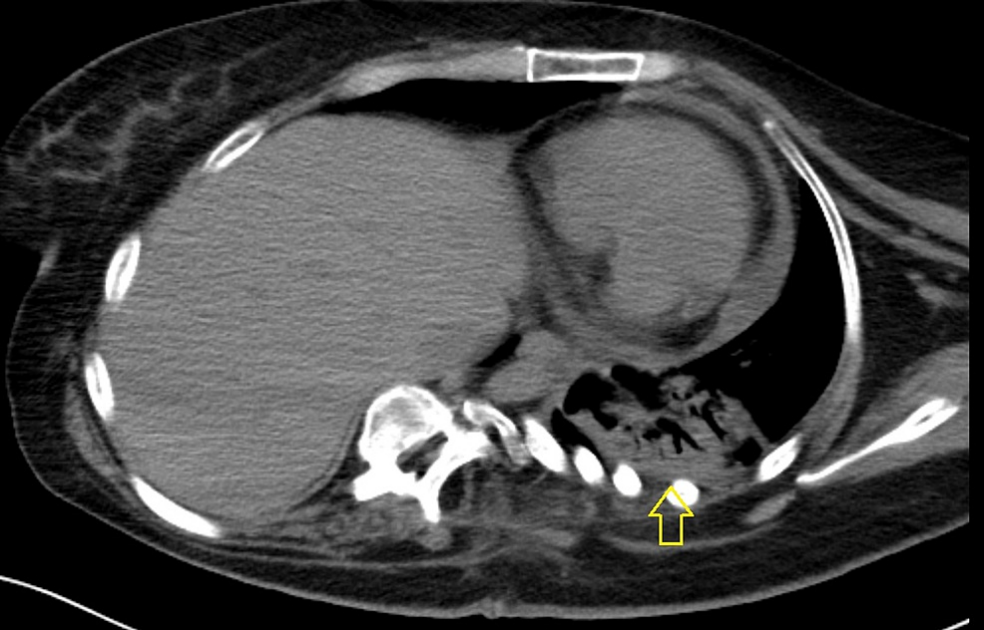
Chest CT scan. A considerable elevation of the left hemidiaphragm with severe dextroconvex scoliosis of the lower thoracic and upper lumbar spine was reported. These findings contributed to severe volume loss in the left hemithorax. Finally, there was compressive atelectasis in the left lower lobe with no evidence of pleural effusion. CT: computed tomography
